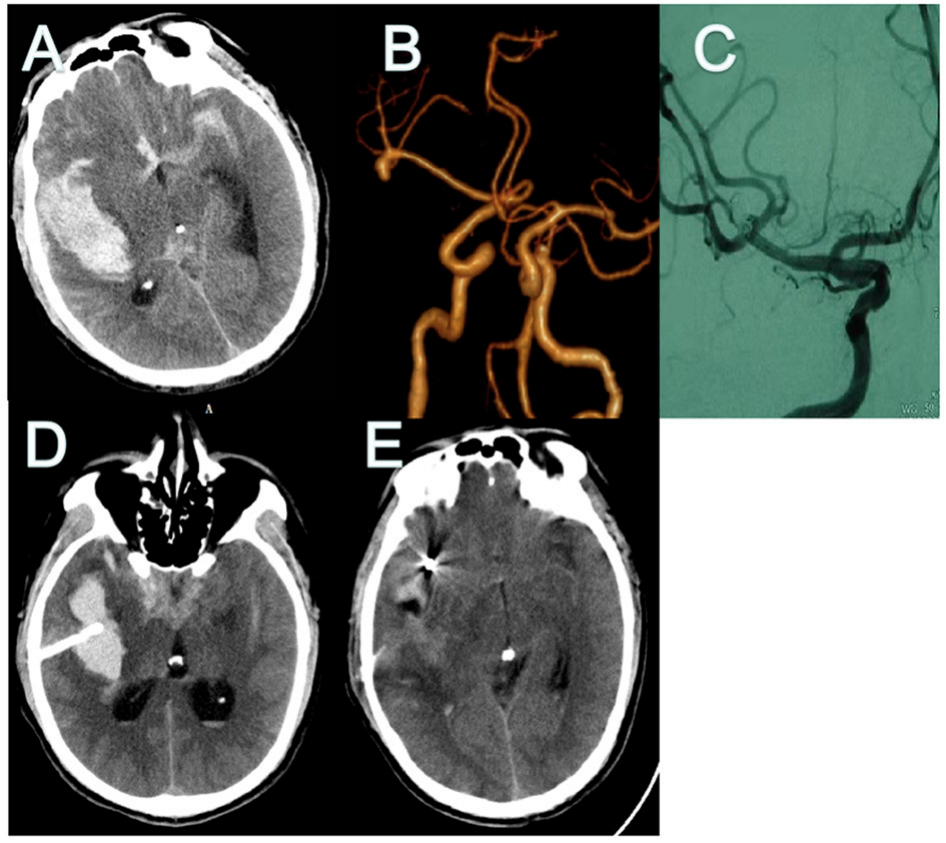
CT and CT angiography in case 1. (A,B) Initial CT scans reveal characteristic findings of ruptured MCA aneurysm with intrasylvian hematoma. Hematoma volume was calculated as 16.5 ml. (C) Post-operative angiography shows the aneurysm is completely occluded and parent artery is unimpeded. (D) Immediate post-operative CT finding the drainage tube was in good position. (E) CT finding Hematoma's almost drained clean on POD 3. CT, computed tomography; MCA, middle cerebral artery; POD, post-operative day.
